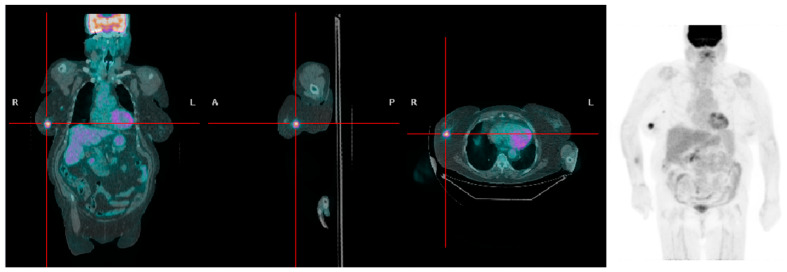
A patient with high BMI presenting with a right-sided breast tumor with high [^18^F]FDG uptake (SUVmax 6.3).
